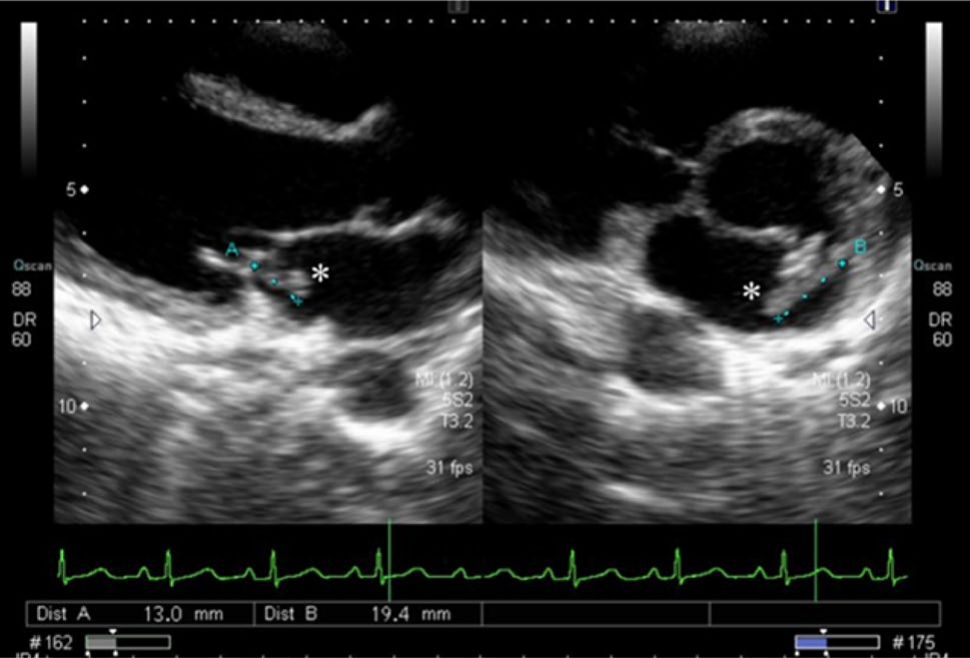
Transthoracic echocardiogram. Left image is long-axis tomogram and right image is short-axis tomogram. There is a large soft vegetation (astreik) on mitral valve
